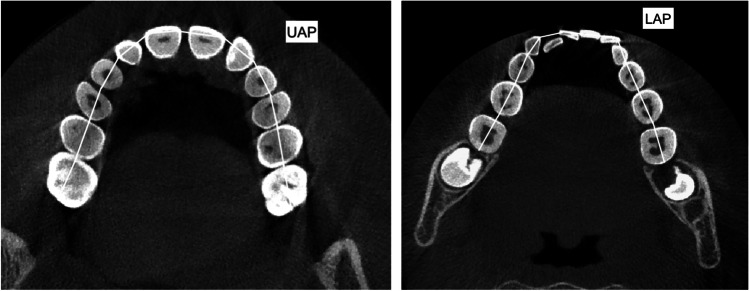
Upper arch perimeter (UAP) and lower arch perimeter (LAP) measurements traced on the CBCTs’ axial section
